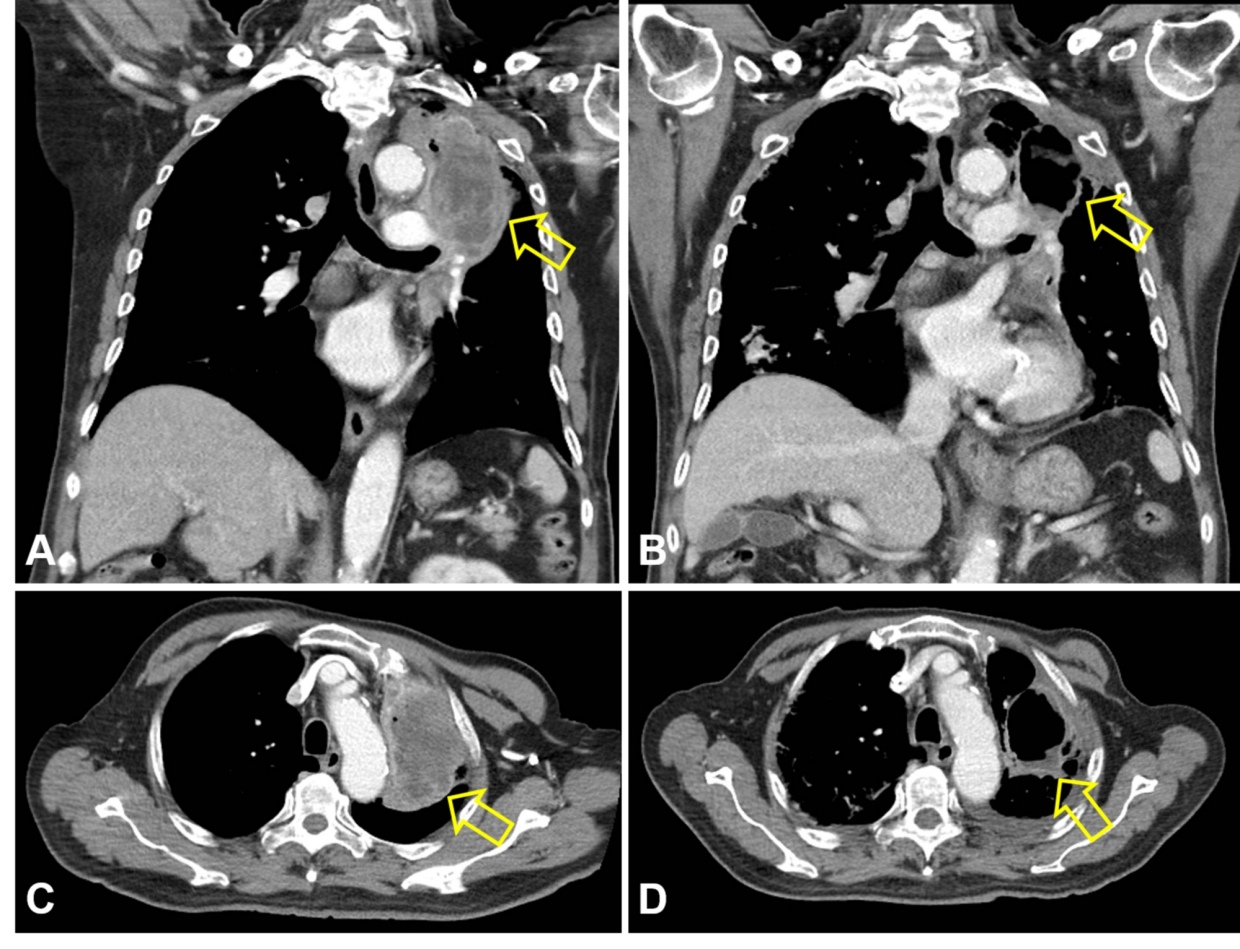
CT scan showing nearly complete necrosis and cavitation of the tumor after therapy: A and C. February 21, 2019. B and D. June 12, 2019 Arrows: Targets of interest CT, computed tomography
